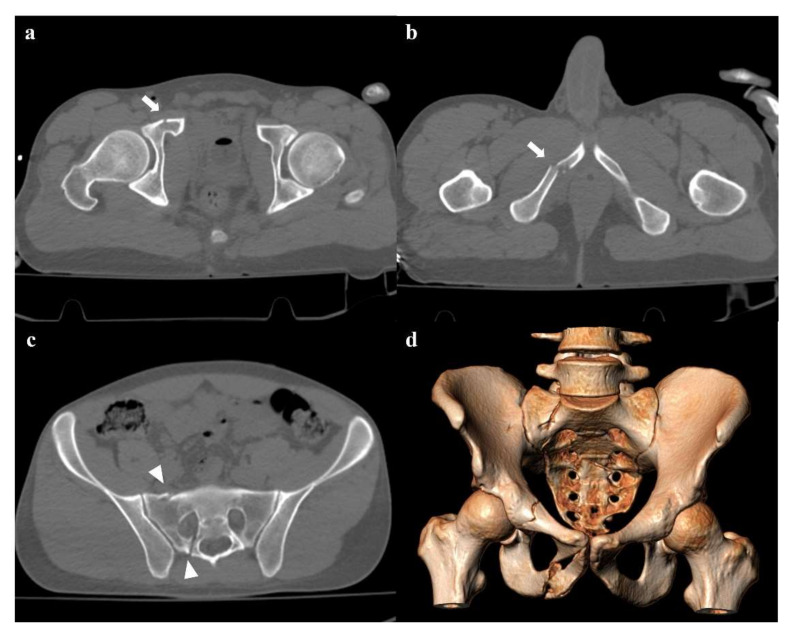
Lateral compression fracture, type 1. Axial computed tomography (CT) images show a fracture of the right-side superior and inferior pubic rami (arrows in (a,b)), and ipsilateral fracture of the sacrum (arrowheads in (c)); the sacral fracture involves the neuroforamina (zone II). Three-dimensional volume-rendering CT reconstruction in anteroposterior (AP) view confirms the type of fracture (d).
